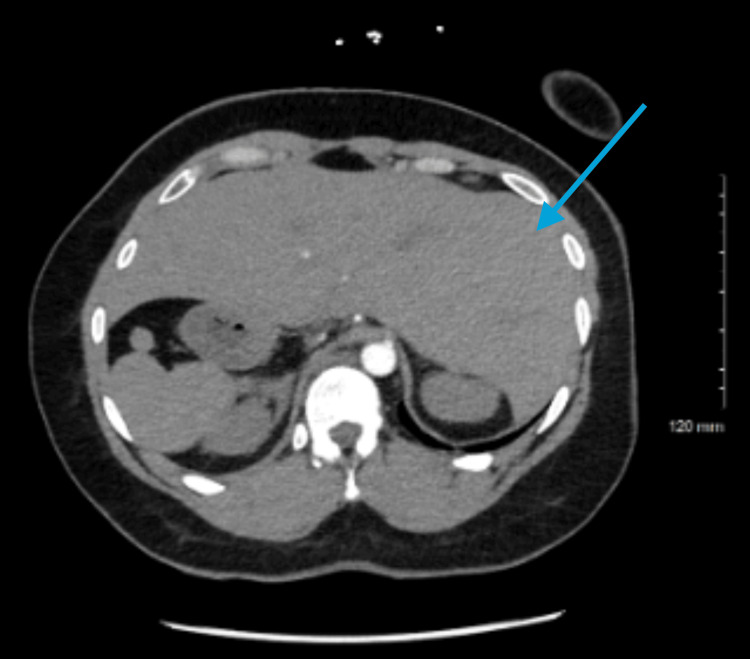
This axial CT image demonstrates features consistent with abdominal heterotaxy most notably multiple splenic nodules clustered within the right upper quadrant reflecting polysplenia. The liver is positioned predominantly on the left producing an atypical visceral arrangement.
